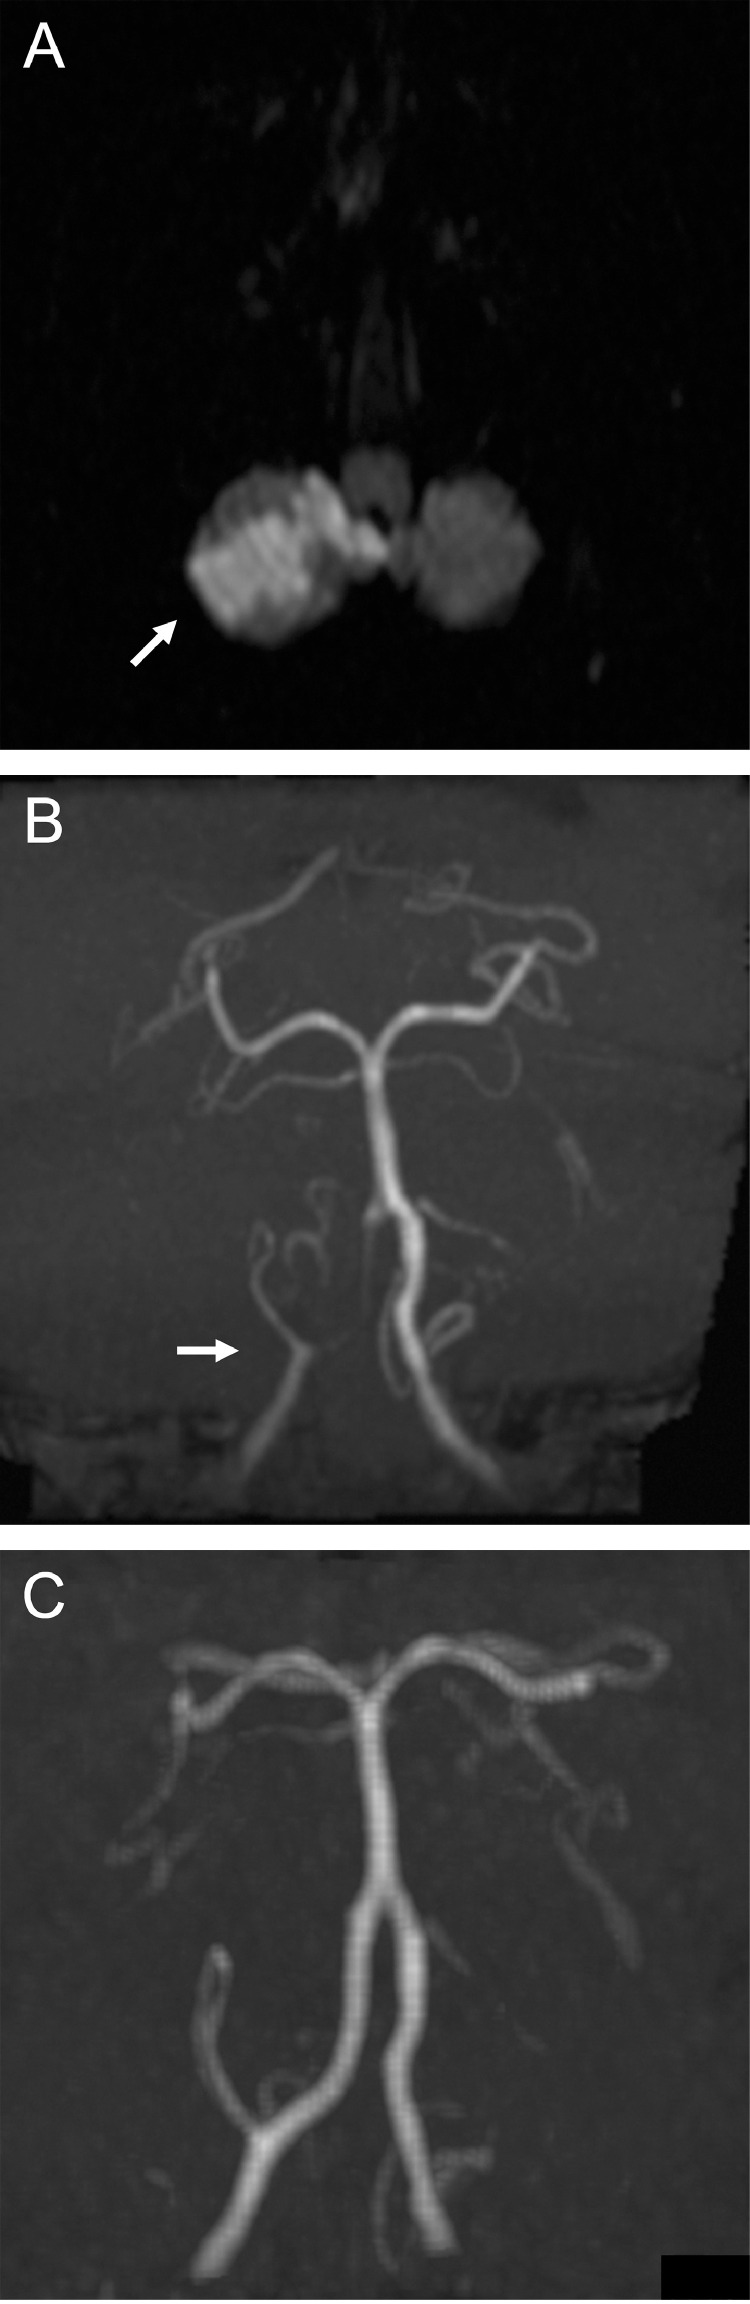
Magnetic resonance imaging showing right cerebellar infarcts (arrow) (A). Magnetic resonance angiography demonstrating impaired delineation of the right vertebral artery (arrow) (B). Adequate delineation had been obtained 1 year previously (C).
